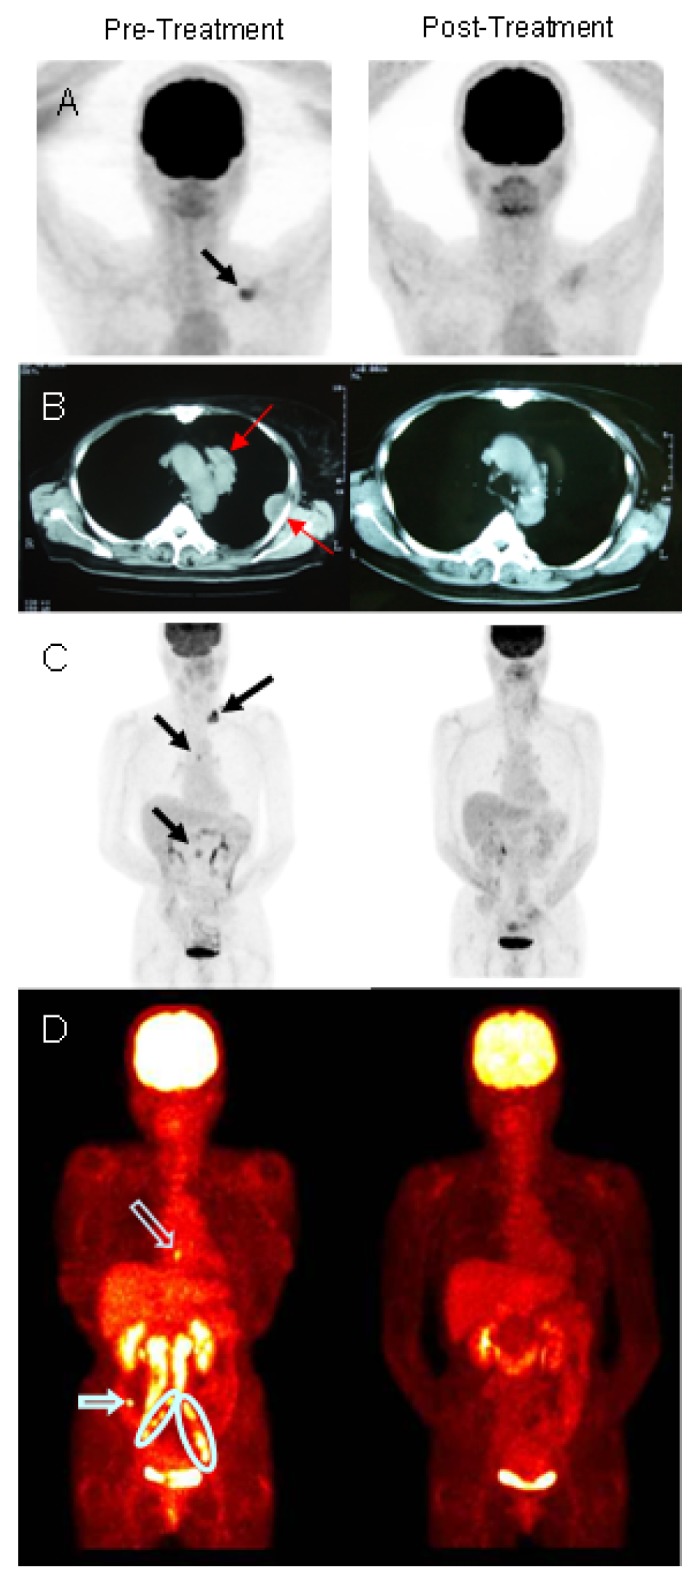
Radiographic evidence of response to treatment. (A–C). Computed tomography (CT) radiographs showing metastatic cancer sites (solid arrows) before and after treatment in two breast cancer and one gastric cancer patient, respectively; (D). PET-CT showing resolution of treated (circled) and un-treated metastatic sites (open arrows) in a patient with cervical cancer. Circled sites were injected with immature dendritic cells (iDC) followed by intensity modulated radiotherapy (IMRT) as per protocol and resolved as were untreated metastatic lesions (open arrows).
